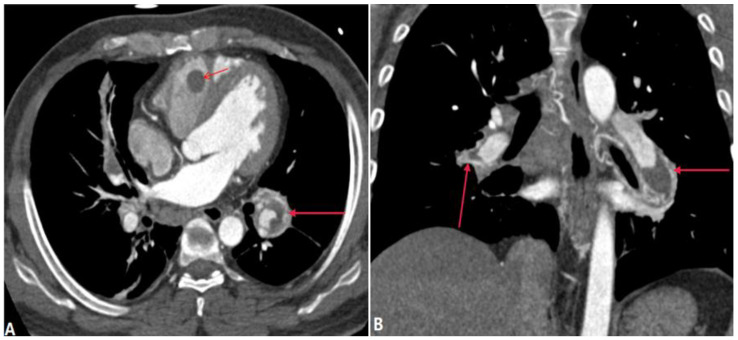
Vasculitis-related pulmonary artery thrombosis. Behcet syndrome (10 years) in a 40-year-old man with hemoptysis. (A,B): Axial and coronal CT MPR images show aneurysmal dilatation of the bilateral lower lobe pulmonary artery with wall thickening and enhancement (long arrows), in situ thrombosis, and stenosis or occlusion. In situ thrombosis can also be found in the right ventricle (short arrow in (A)). MPR—multiplanar reformation.
